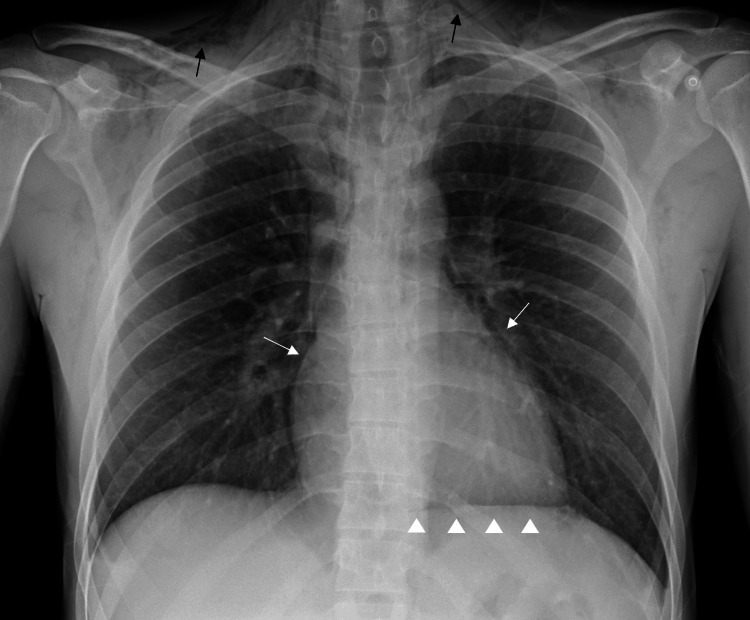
Chest radiograph An anteroposterior radiograph chest view shows a sharp line of lucency outlining the left and right heart borders (white arrows); mediastinal air between the heart and the superior surface of the diaphragm (“partial” diaphragm sign denoted by arrowheads); and subcutaneous emphysema in the supraclavicular and neck areas (black arrows).
